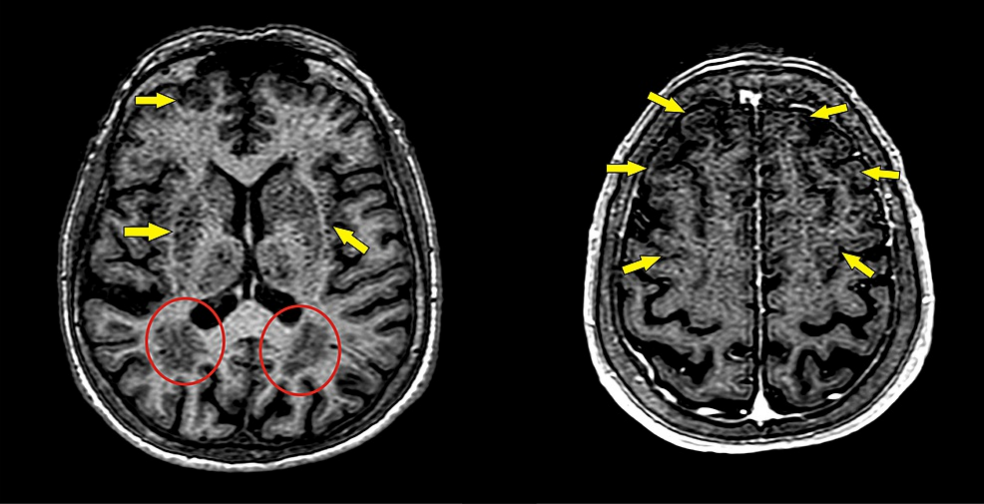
Brain MRI Brain 3D T1-weighted Magnetic Resonance Imaging with contrast media showing lesions of chronic cerebrovascular disease. Notice the areas of white matter hyperintensity (highlighted with red circles) and multiple lacunar infarcts in the thalamus and the frontal lobes (pointed with yellow arrows).
